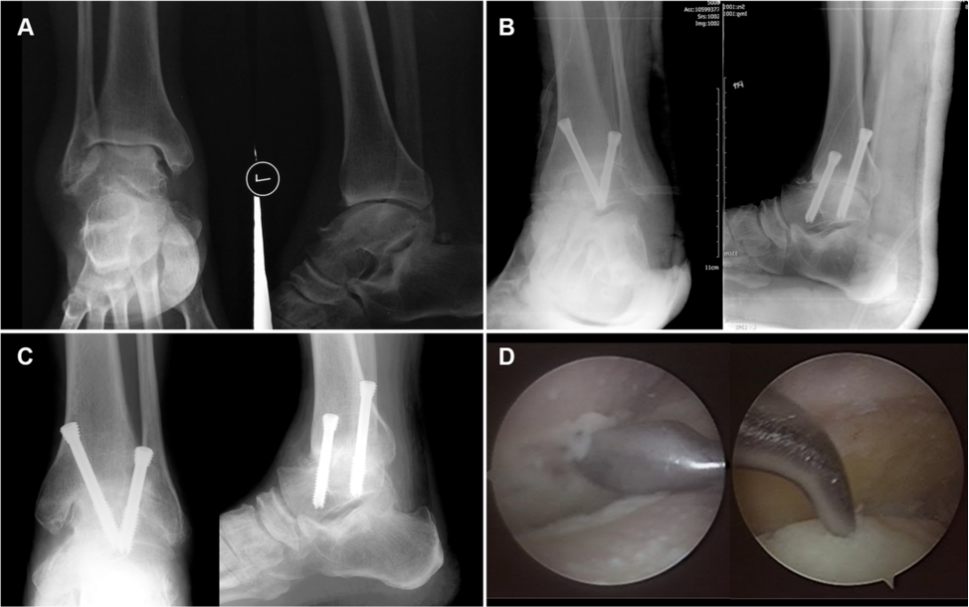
Case 2. a Preoperative radiographs. b Radiographs at 1 day postoperatively. c Radiographs at 12 days postoperatively. d Arthroscopic surgery in progress
